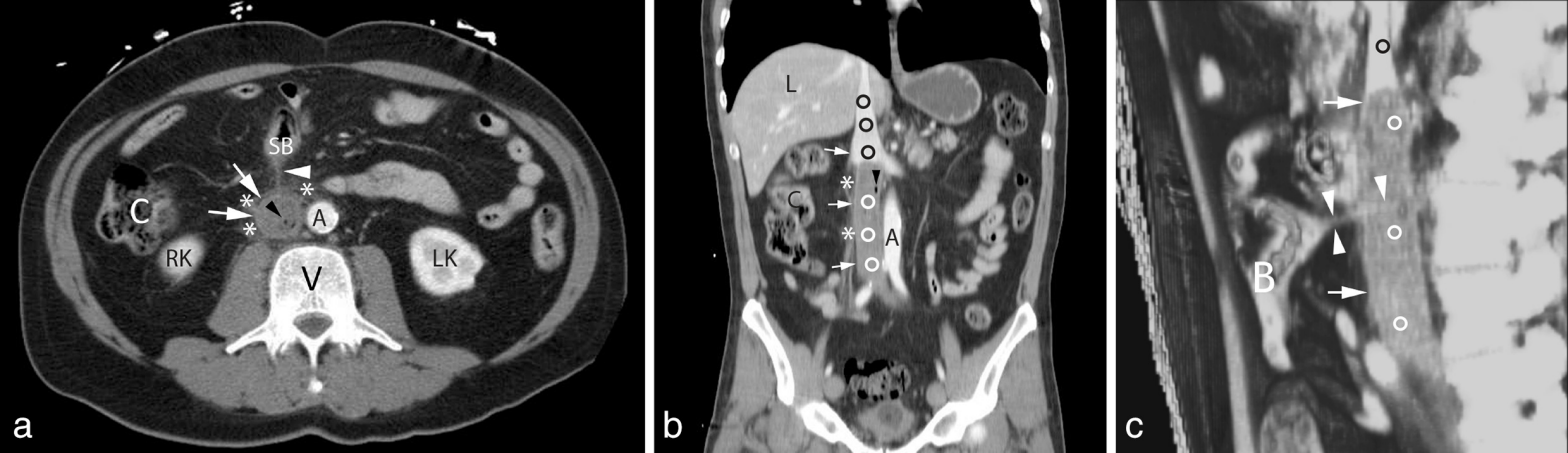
Contrast-enhanced CT scan of the abdomen showing small bowel perforation and inferior vena cava septic thrombosis caused by an accidentally ingested toothpick. (a) Axial image shows a complete thrombosis of the inferior vena cava (arrows) with some air bubbles within (black arrowhead). Perivascular phlogistic fat thickening can also be seen (asterisks). The toothpick can be appreciated as a thin linear image (white arrowhead) connecting the inferior vena cava and a small bowel loop (SB). (b) Coronal reformatted image confirms that the inferior vena cava (arrows) is partially occupied by a septic thrombus (white circles), containing a small air bubble (black arrowhead), surrounded by perivascular phlogistic fat thickening (asterisks). Note that the proximal inferior vena cava is patent (black circles). (c) A maximum intensity projection sagittal reformat shows the toothpick (white arrowheads) connecting a small bowel loop (B) with the inferior vena cava, in which a thrombus is present (white circles). Perivascular fat is thickened due to phlogosis (arrows). Note that the proximal inferior vena cava is patent (black circle). A, aorta; C, colon; L, liver; LK, left kidney; RK, right kidney; V, vertebral body.
